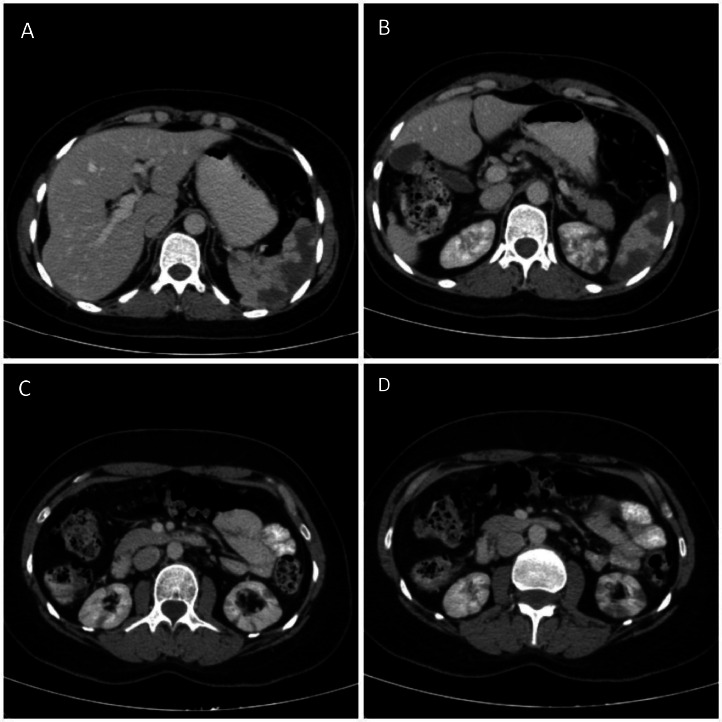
(A-D) Axial images of abdominopelvic CT scan with contrast show nonenhancing hypodense wedge-shaped areas in the spleen and both kidneys that favor multiple infarcts. Also, mild atrophy of the right liver lobe is depicted, secondary to autoimmune hepatitis.
